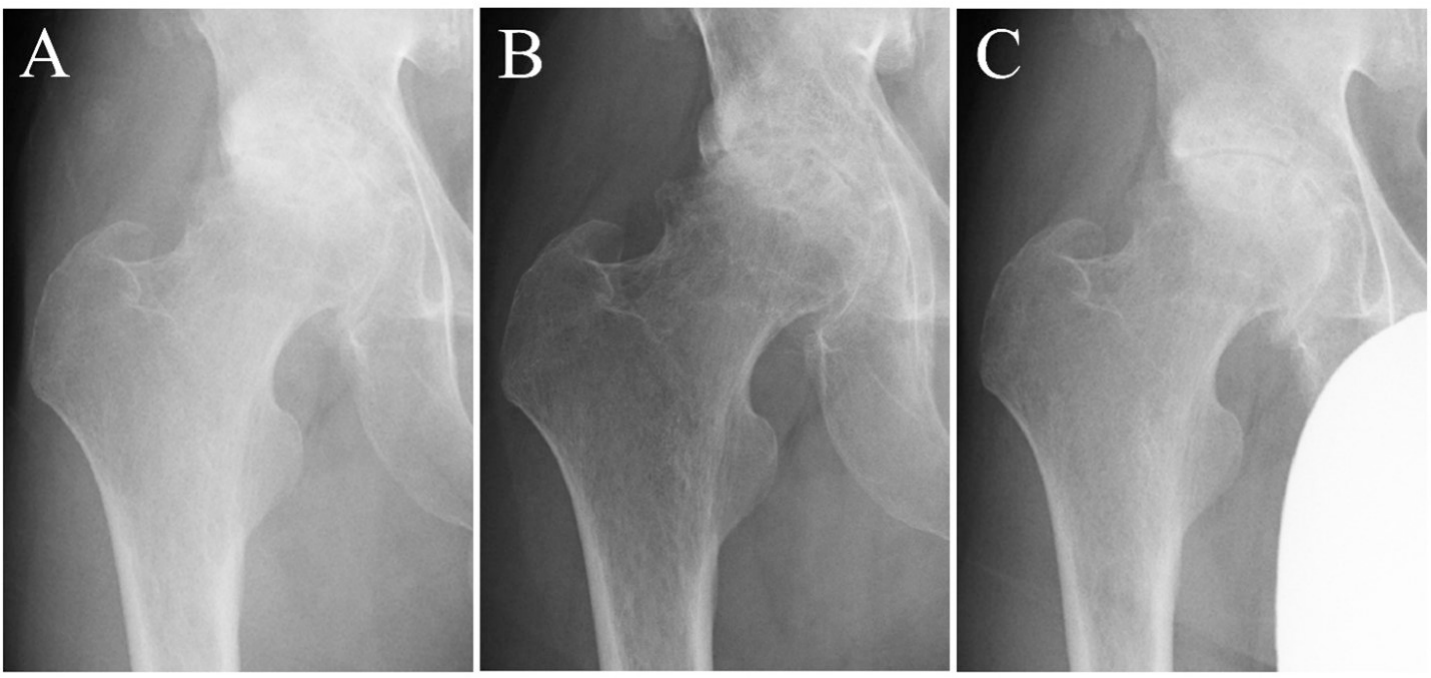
Recording from a single subject (patient A) showing radiographic changes in hip joint. At hospital admission (A), discharge (B), and at the 6-month post-discharge checkup (C).
